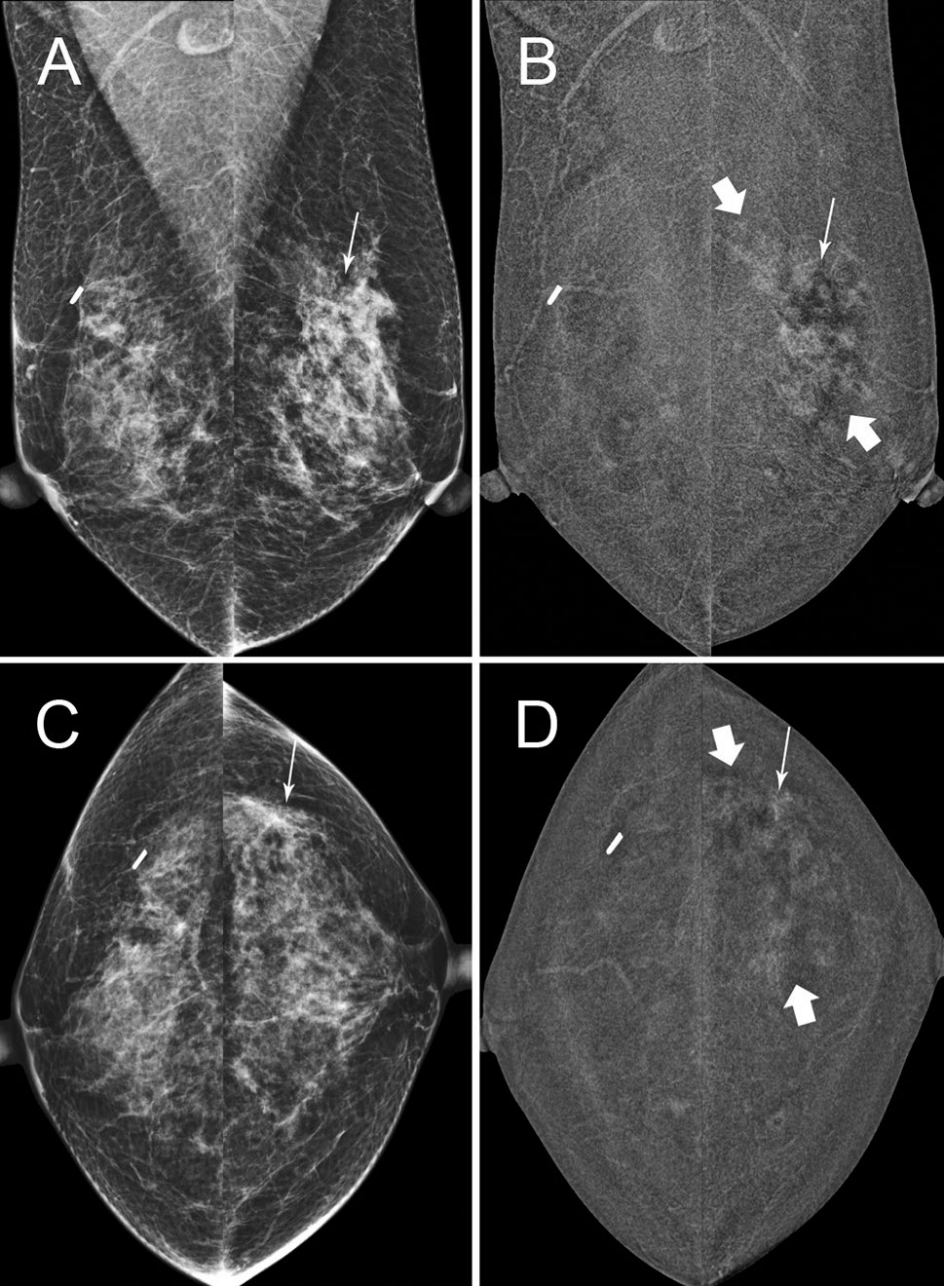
A and C, 53 year-old patient, 2D 2019 surveillance images, MD BIRADS C. She had right wide local excision 2015 for 20 mm Intermediate grade DCIS and excision of papilloma with atypia on the left in the upper outer quadrant at the same time. Thin arrow on left is site of scar, stable on tomography. No other abnormalities on 2D,3D or ultrasound on the left and no calcifications. B and D are C + images from the same surveillance study showing extensive non-mass enhancement (thick arrows) throughout the left upper outer quadrant and mild BPE bilaterally. Stereotactic biopsy targeted to the enhancing scar (thin arrow) in the left upper outer quadrant was performed. Mastectomy showed 9 cm intermediate grade DCIS without invasion. SNB negative. This is an example of a contrast-only lesion
